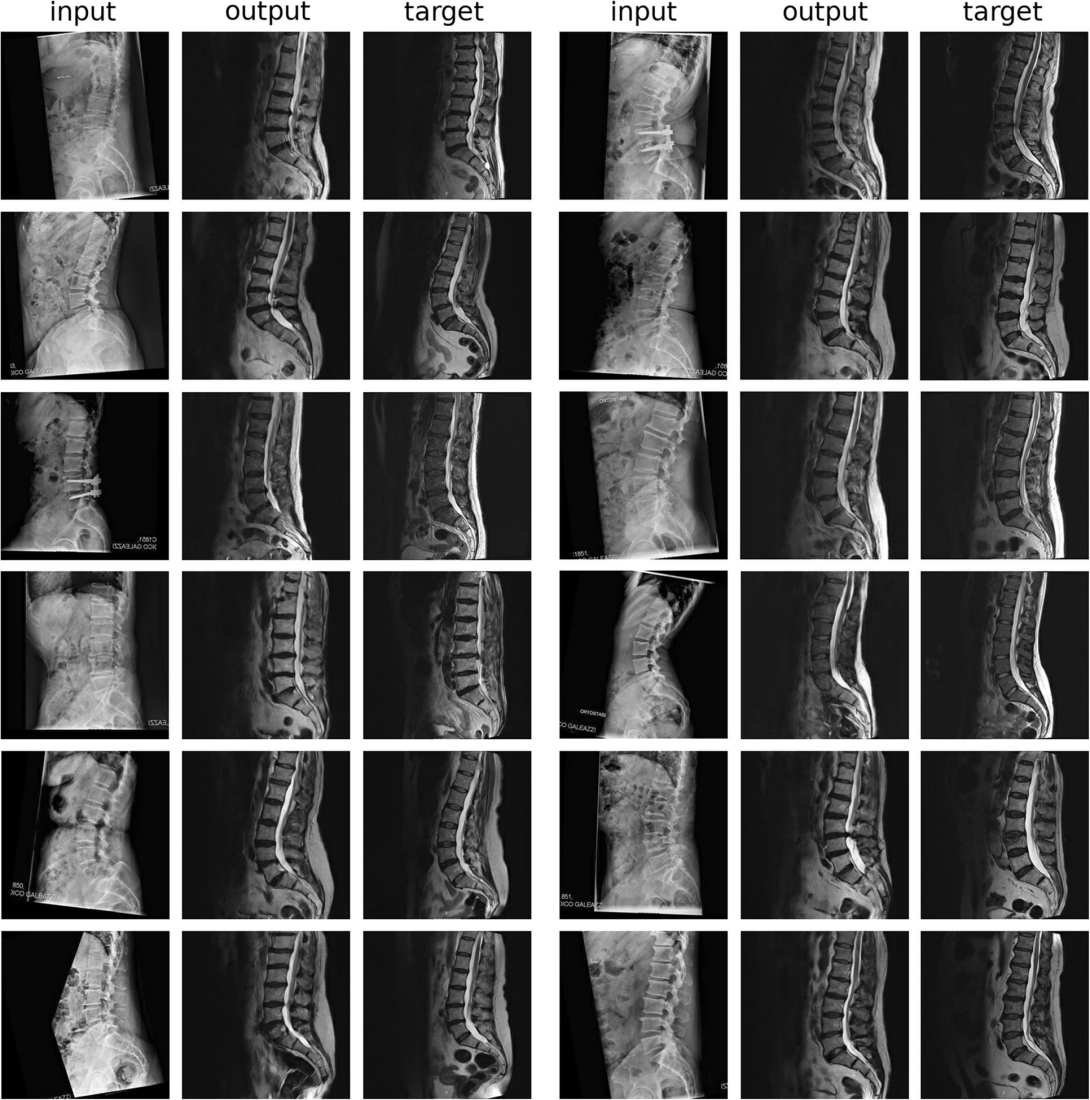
Representative results (12 patients) of the translation from sagittal x-ray projections to T2W midsagittal MRI scans. input: original x-ray image, registered to match the alignment of the original MRI; output: synthetic T2W midsagittal MRI; target: original T2W midsagittal MRI. A few gross errors in the anatomy can be identified: the L1 in the first and fourth patients on the *left*; the sacrum in the first patient on the *right*; the thoracolumbar junction in the third patient on the *right*; L1 and the sacrum in the fourth patient on the *right*
